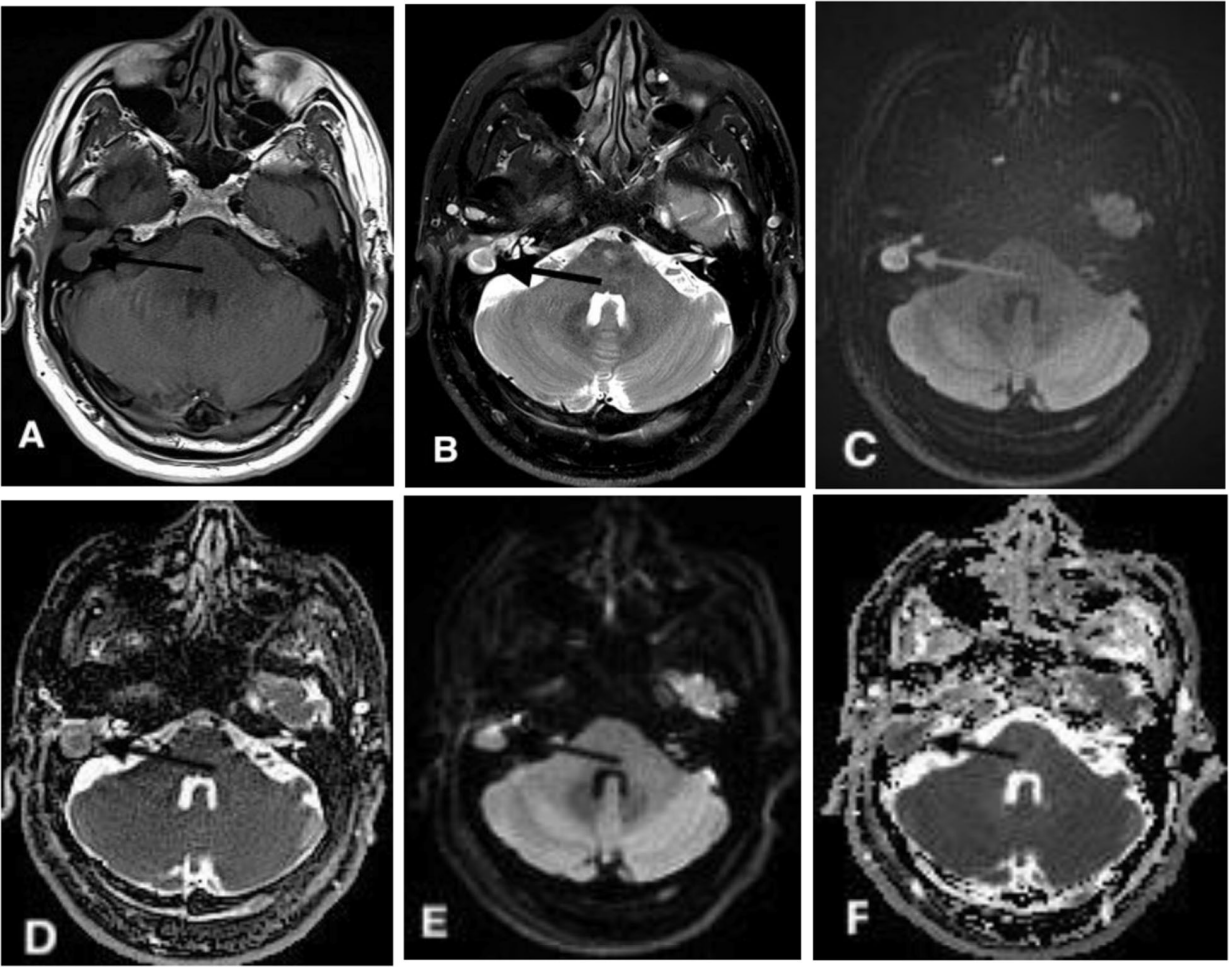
A 51-year-old male with primary cholesteatoma in the right middle ear confirmed by right mastoidectomy. Axial T1WI (a) and T2WI (b) showed the anatomical location of the cholesteatoma (arrow) in the right middle ear. c, e: TGSE BLADE (b1000) and RESOLVE (b1000) DWI showed a restricted diffusion lesion (high signal) in the right mastoid bone. d, f: The ADC on TGSE BLADE and RESOLVE DWI was 0.689 × 10^− 3^ mm^2^/s and 0.791 × 10^− 3^ mm^2^/s, respectively. However, the cholesteatoma lesion (arrow) was not as clear on RESOLVE DWI as on TGSE DWI. Moreover, the structures of the nasal cavity were obviously distorted on the RESOLVE b1000 (e) and ADC (f) maps, whereas almost no distortion was observed on the TGSE b1000 and ADC maps. Images (c and e) show the geometric deformation values of the entire image for images with a score of 4 (no distortion) and 2 (moderate distortion)
